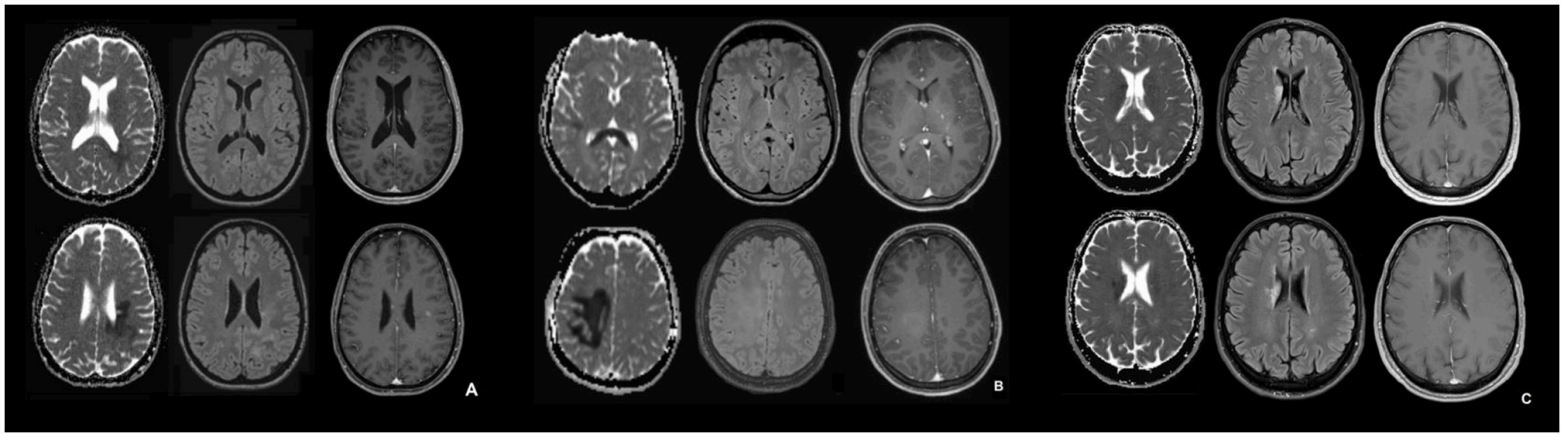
MRI in the acute phase of NMOSD (A), tumefactive MS (B), and ischemic stroke (C). (A–C): Apparent diffusion coefficient (ADC) map (left), transverse FLAIR-attenuated inversion recovery weighted scan (middle), transverse contrast-enhanced T1 weighted scan (right) for the NMOSD patient (A), the patient with tumefactive MS (B) and the patient with ischemic stroke (C). In (B) the contrast enhancement in basal ganglia on the left corresponds to a developmental venous anomaly.
